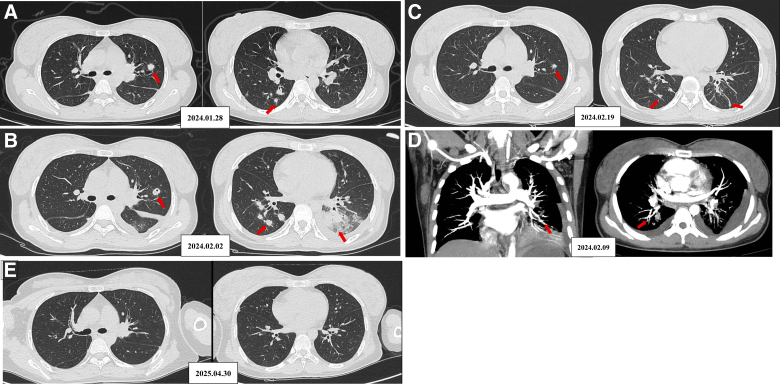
Temporal evolution of chest CT imaging findings. (A) shows large consolidation with peripheral inflammatory exudation in both lower lobes (January 28, 2024). (B) Left pneumonia progression more than before, with multiple cavitary lung nodules (February 2, 2024) (indicated by arrows). (C) Left lower lobe pneumonia was significantly absorbed more than (B) (February 19, 2024). (D) CTPA indicated microthrombi in the bilateral lower lobe pulmonary arteries (February 9, 2024) (indicated by arrows). (E) Follow-up chest CT on April 30, 2025, after regular outpatient treatment of the BD, showing near-complete resolution of the bilateral pulmonary opacities.
